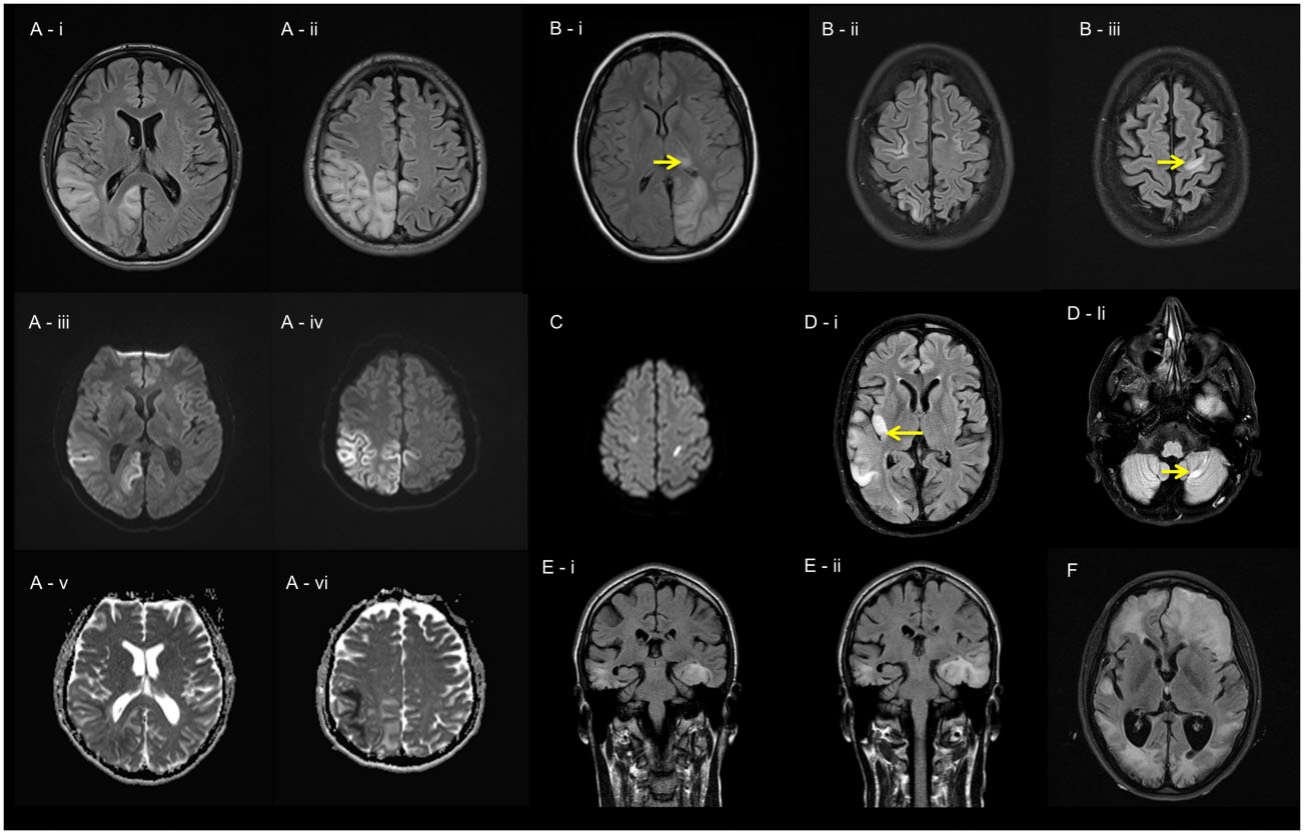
Stroke-like lesions identified on MRI head. Fluid-attenuated inversion recovery (FLAIR) sequence shows characteristic right occipital, temporal, parietal [A(i)] and left parietal [A(ii)] changes with restricted diffusion [A(iii and iv)] and mixed apparent diffusion coefficient (ADC) map changes [A(v and vi)] in a 20-year-old male with the m.3243A>G variant presenting with encephalopathy, occipital seizures, left hemianopia and apraxia. FLAIR sequence shows signal abnormalities involving left occipital lobe and pulvinar nucleus [B(i)] and multiple cortical signal abnormalities with restricted diffusion [B(ii and iii)] in a 25-year-old female with recessive *POLG* variants presenting with right hemianopia and status epilepticus. (C) Diffusion-weighted imaging identifies bilateral signal abnormalities approximate to precentral gyri in a 16-year-old female with recessive *POLG* variants presenting with refractory epilepsia partialis continua. FLAIR sequence demonstrates the phenomenon of cross-cerebellar diaschisis where signal abnormalities are present in right temporal and insular cortices [D(i)] and contralateral cerebellar hemisphere [D(ii)]. Coronal view of FLAIR sequence demonstrates bilateral temporal lobe changes [E(i)] at baseline, and the signal abnormalities in left mesial temporal lobe (hippocampus) evolved to involve the remaining temporal lobe on the interval scan a week later [E(ii)] in a 34-year-old male with the m.3243A>G variant presenting with headache, right hemianopia, global aphasia and focal motor seizures. Multiple, confluent signal abnormalities identified in both cerebral hemispheres (F) in a 24-year-old female with the m.3243A>G variant presenting with recurrent stroke-like episodes; only the signal abnormalities of left frontal lobe are associated with restricted diffusion (not shown) and there is evidence of generalized brain atrophy.
